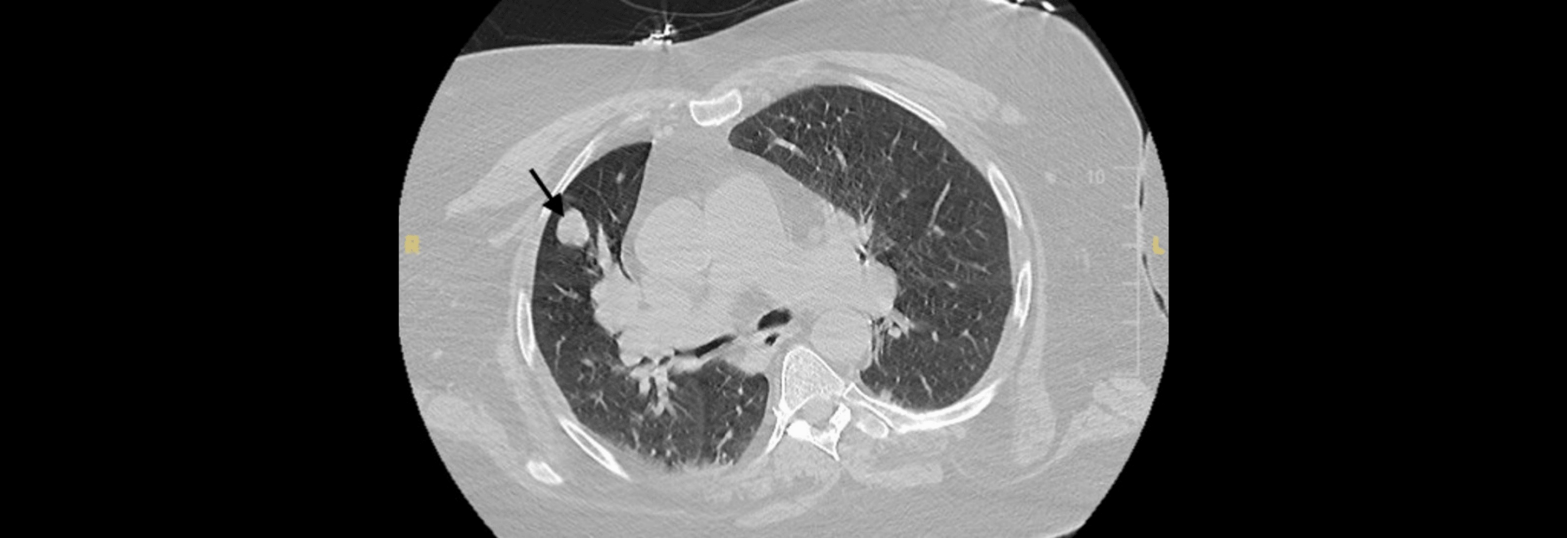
CT of the chest showing right upper lobe nodule (arrow) CT: computed tomography
